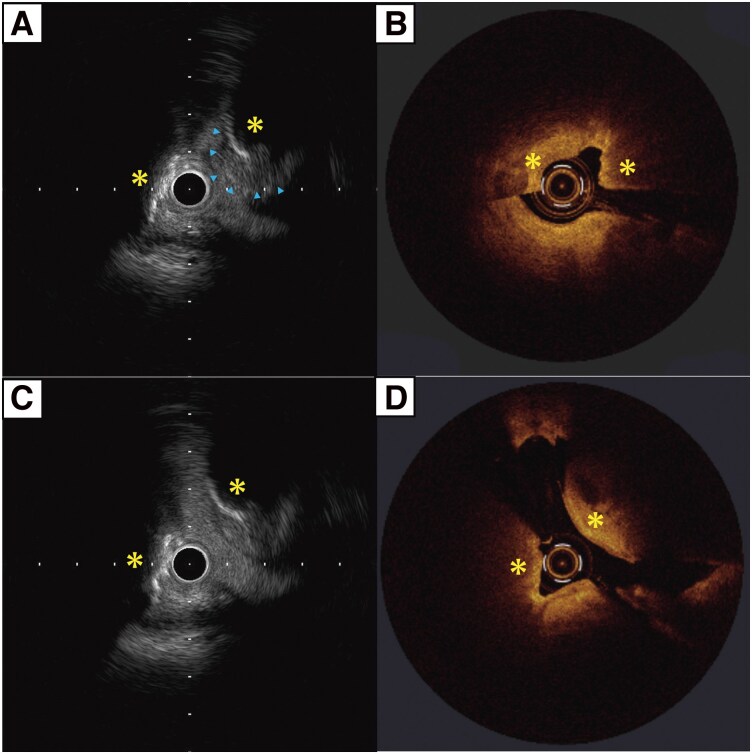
(*A*, *B*) Index ACS event: intravascular ultrasound (IVUS) and optical coherence tomography (OCT) images demonstrating calcified nodules (CNs) (yellow asterisks) with overlying thrombus (blue arrowheads). (*C*, *D*) Follow-up (one week after intensive antithrombotic therapy): repeat IVUS and OCT at the same site, showing persistent CNs (yellow asterisks) with thrombus resolution.
